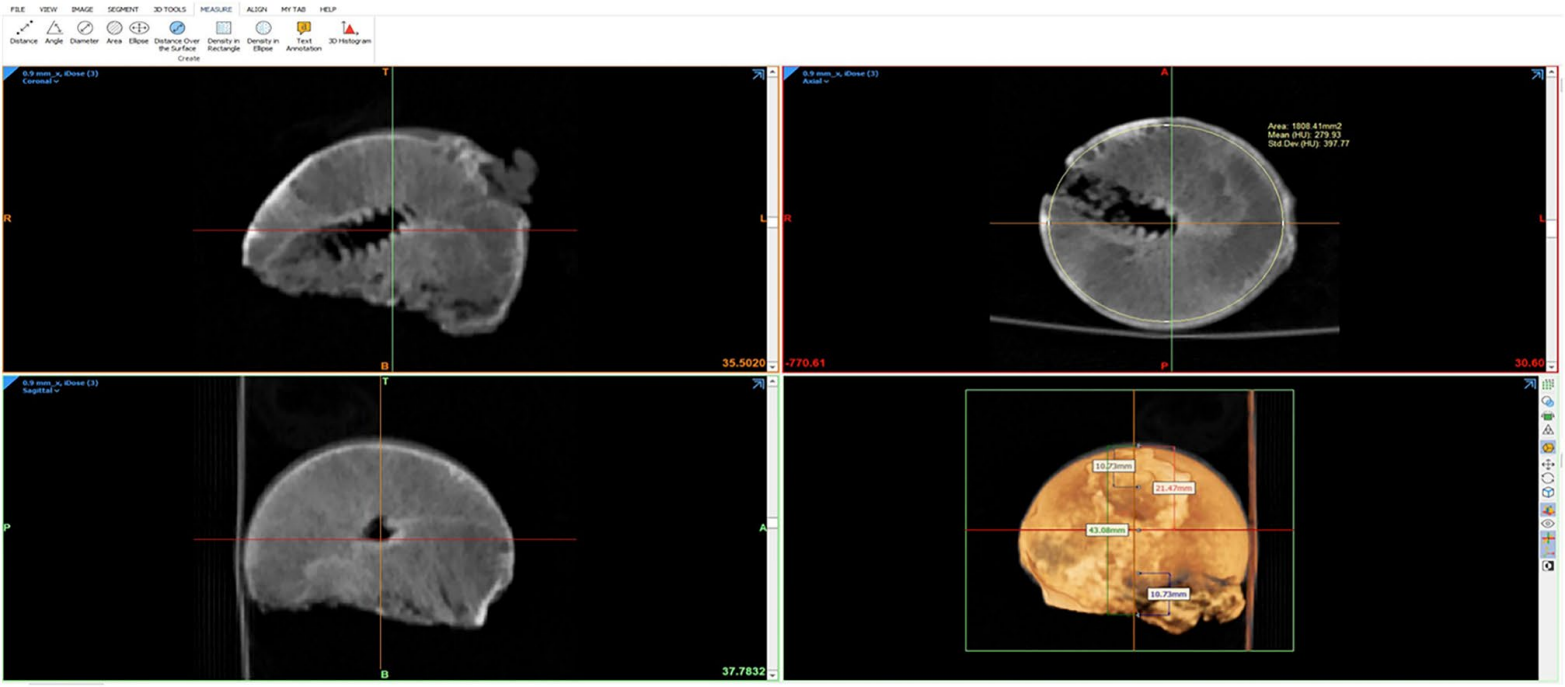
Reconstruction of CT-scans
